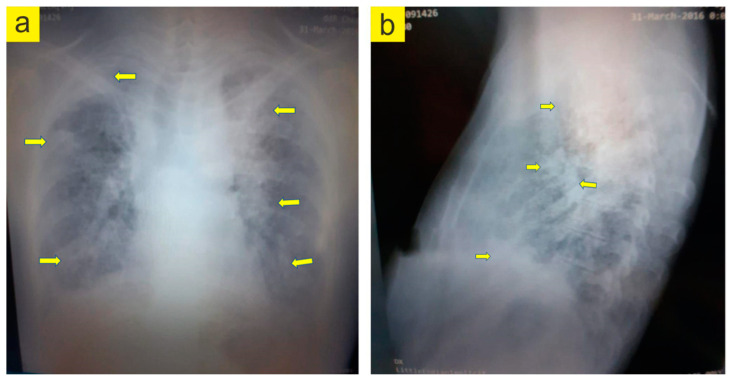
Chest radiography. (a). PA lung grapy. (b). Lateral lung grapy. Yellow arrows indicate the problem areas. (Images archive of the first author).
